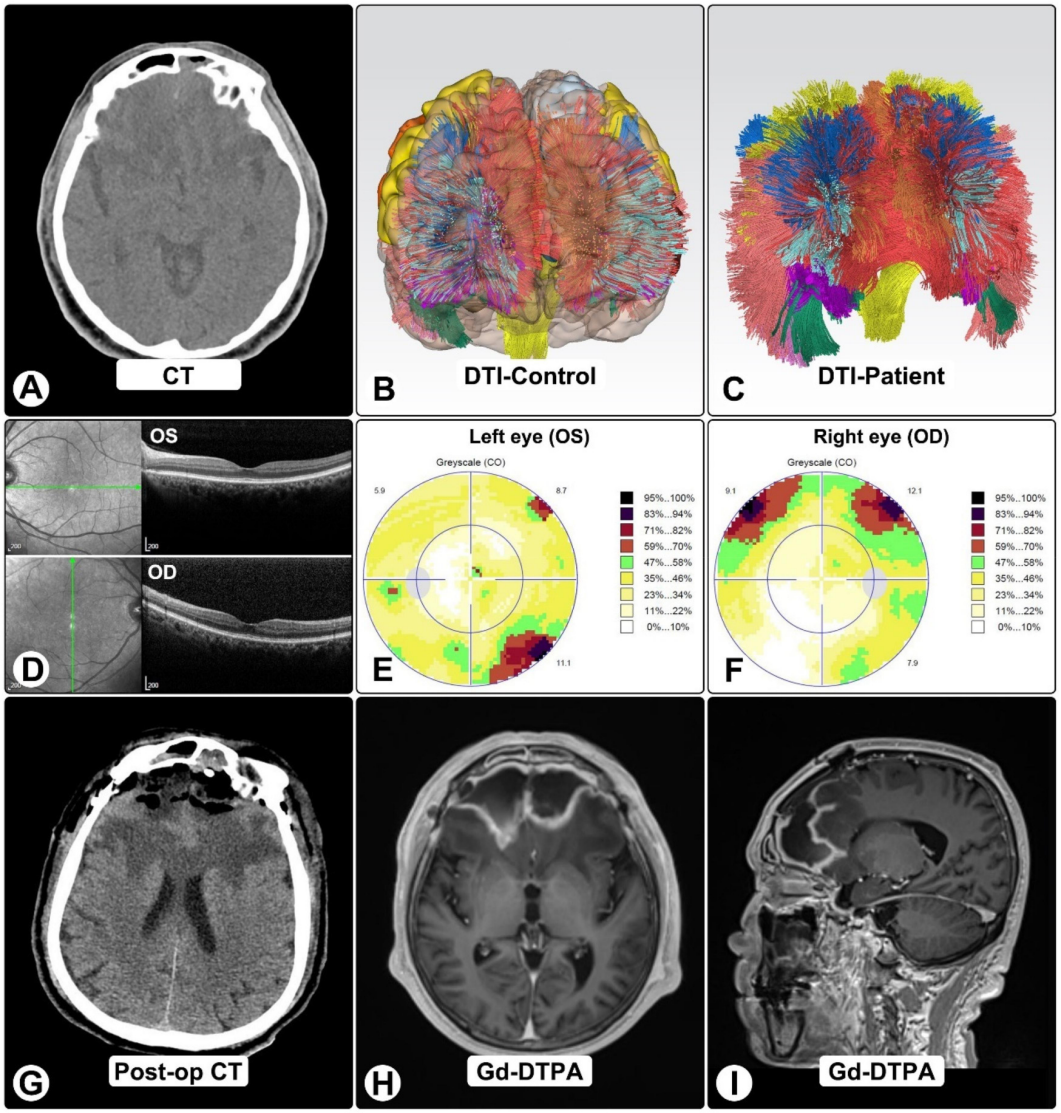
DTI tractography, OCT, and visual findings. (A) Pre-op CT shows bilateral frontal lesions with vasogenic edema and mass effect. (B,C) DTI tractography in normal brain (B) and patient (C), showing marked frontal tract loss, including bilateral anterior thalamic radiations, genu of corpus callosum, cingulum bundles, and forceps minor. (D) OCT imaging. (E,F) Octopus visual fields show right superior altitudinal defect (E) and left inferotemporal scotoma (F), consistent with compressive optic neuropathy. (G) Post-op CT showing expected changes after craniotomy and abscess evacuation. (H,I) One-month post-op MRI shows residual ring-enhancing lesions with reduced size and mass effect.
